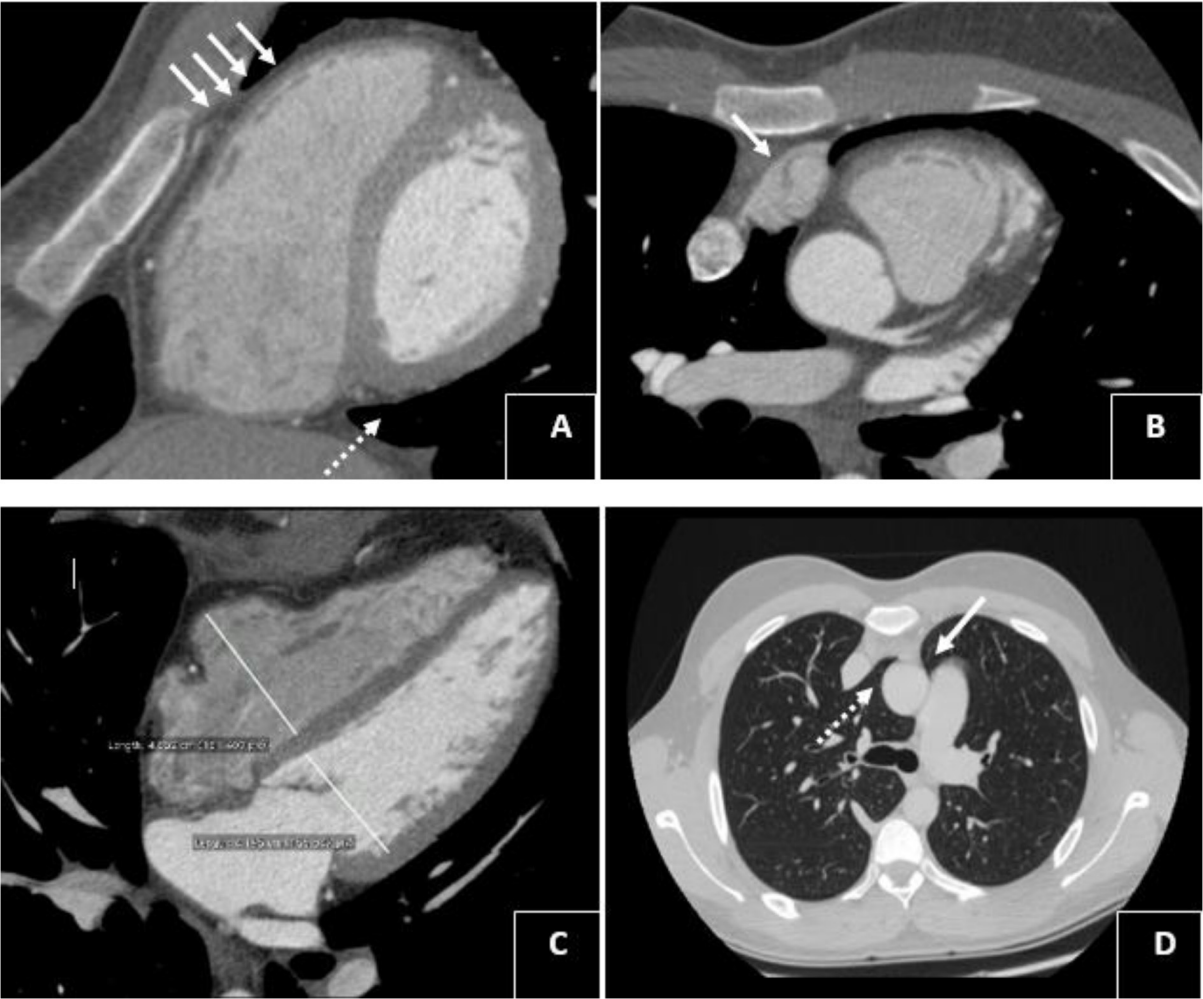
Cardiac CT demonstrating (A) the partial non-visualization of the right
